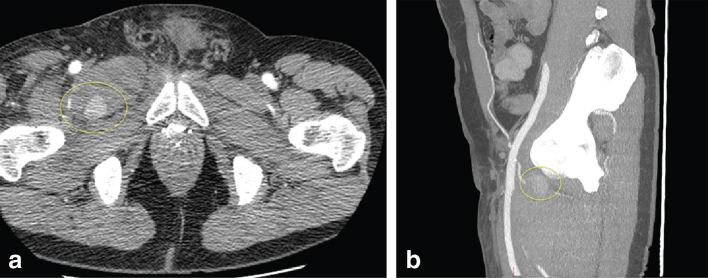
(a, b) Axial and sagittal slices of a CT angiogram showing depth of the pseudoaneurysm.
